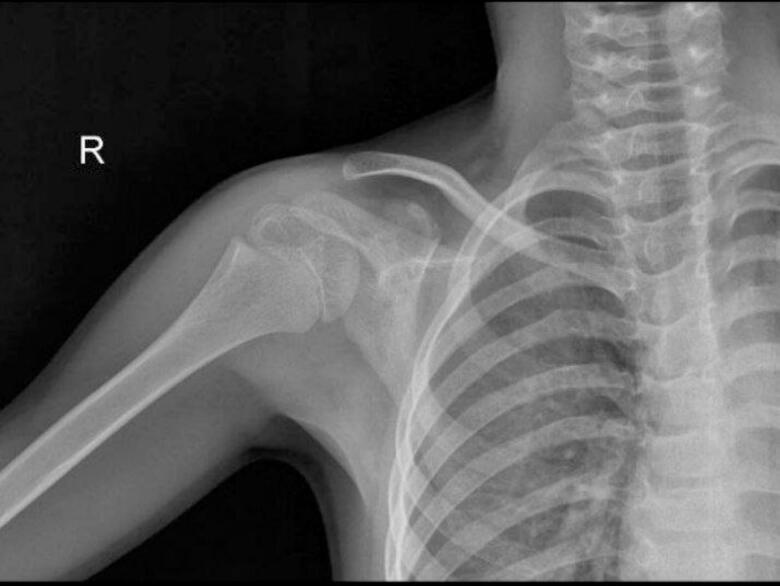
Post-op X-ray showing the result of the healing after 24 months.
